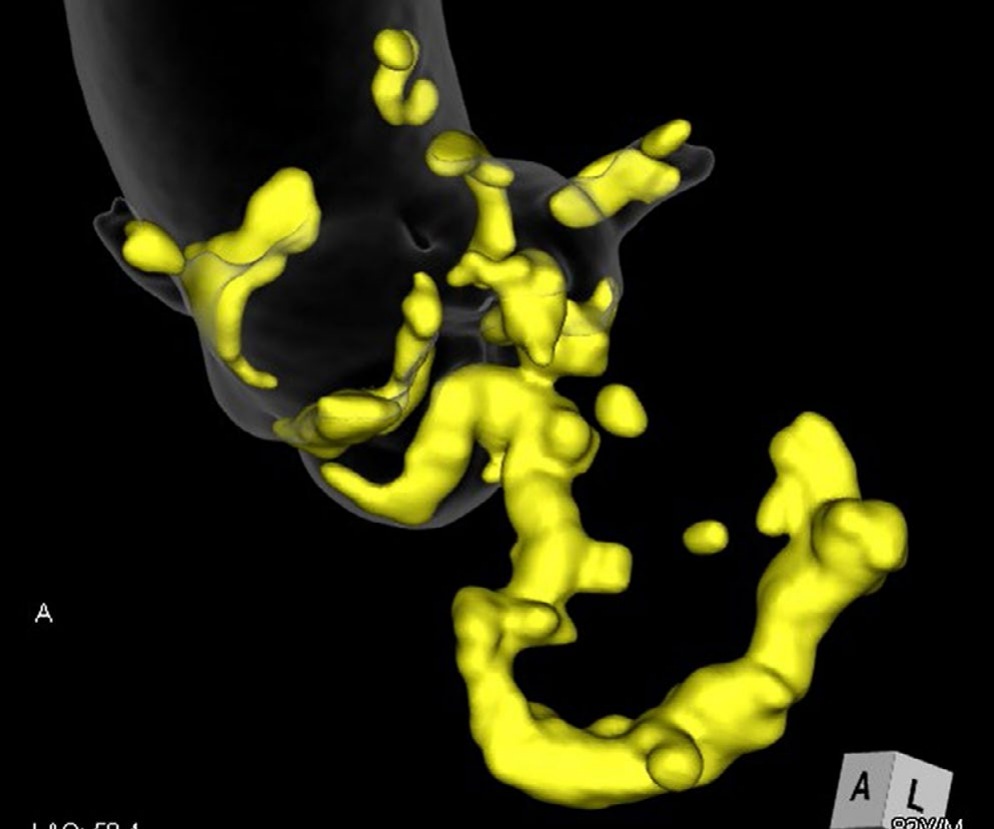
Preoperative three‐dimensional reconstructed computed tomography images. Severe calcifications ranged continuously from the noncoronary cusp to the left ventricular outflow tract and mitral annulus. Mitral annular calcification was observed in three‐fourths circumference (half of the anterior leaflet and entire posterior leaflet)
